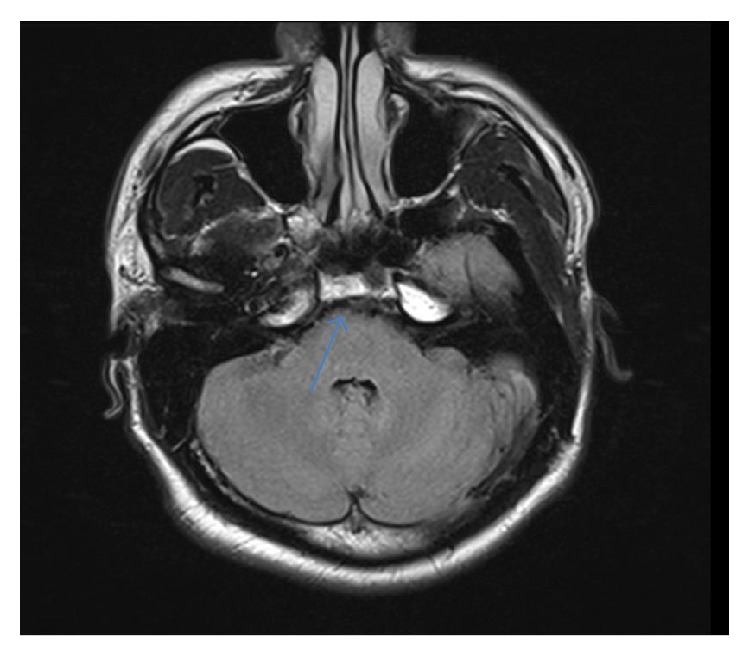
MRI of brain T1 axial + gadolinium with resolution of previously visualized enhancing lesion, after anti-TB treatment.
